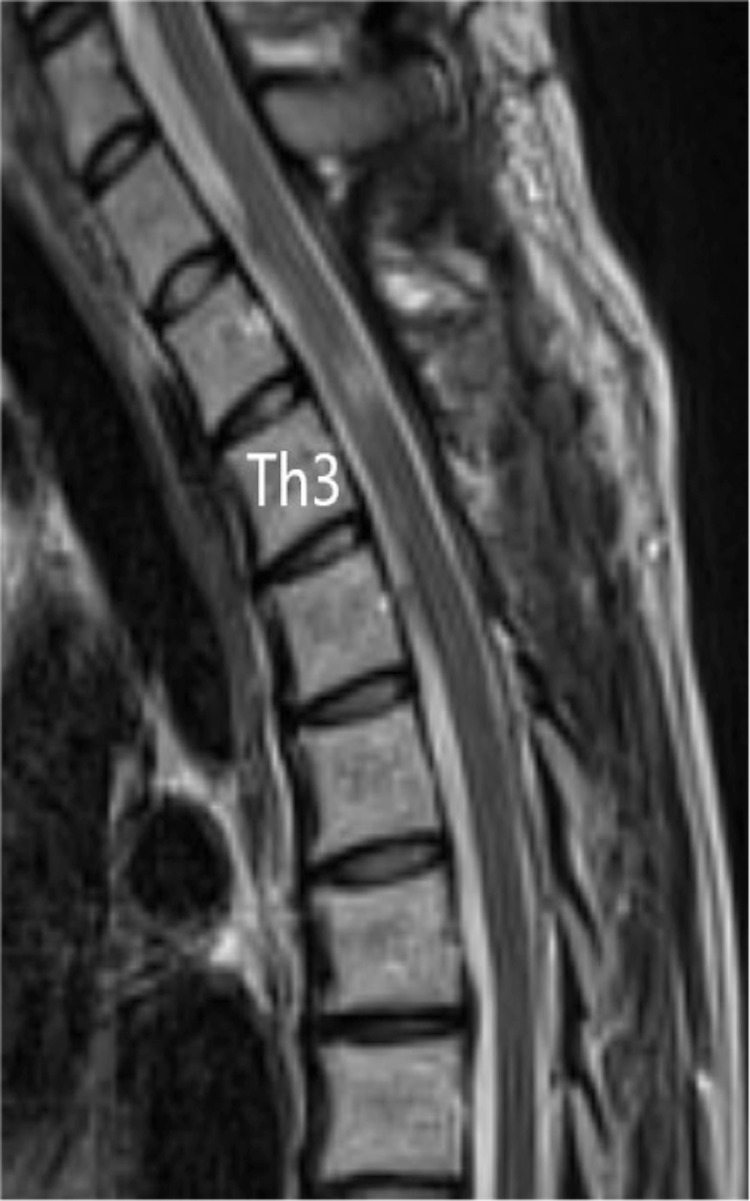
Sagittal T2-weighted MR images 12 weeks after the operation The high-intensity region in the spinal cord of the Th2-weighted image at the higher position of Th3 remained, but the hematoma disappeared.
